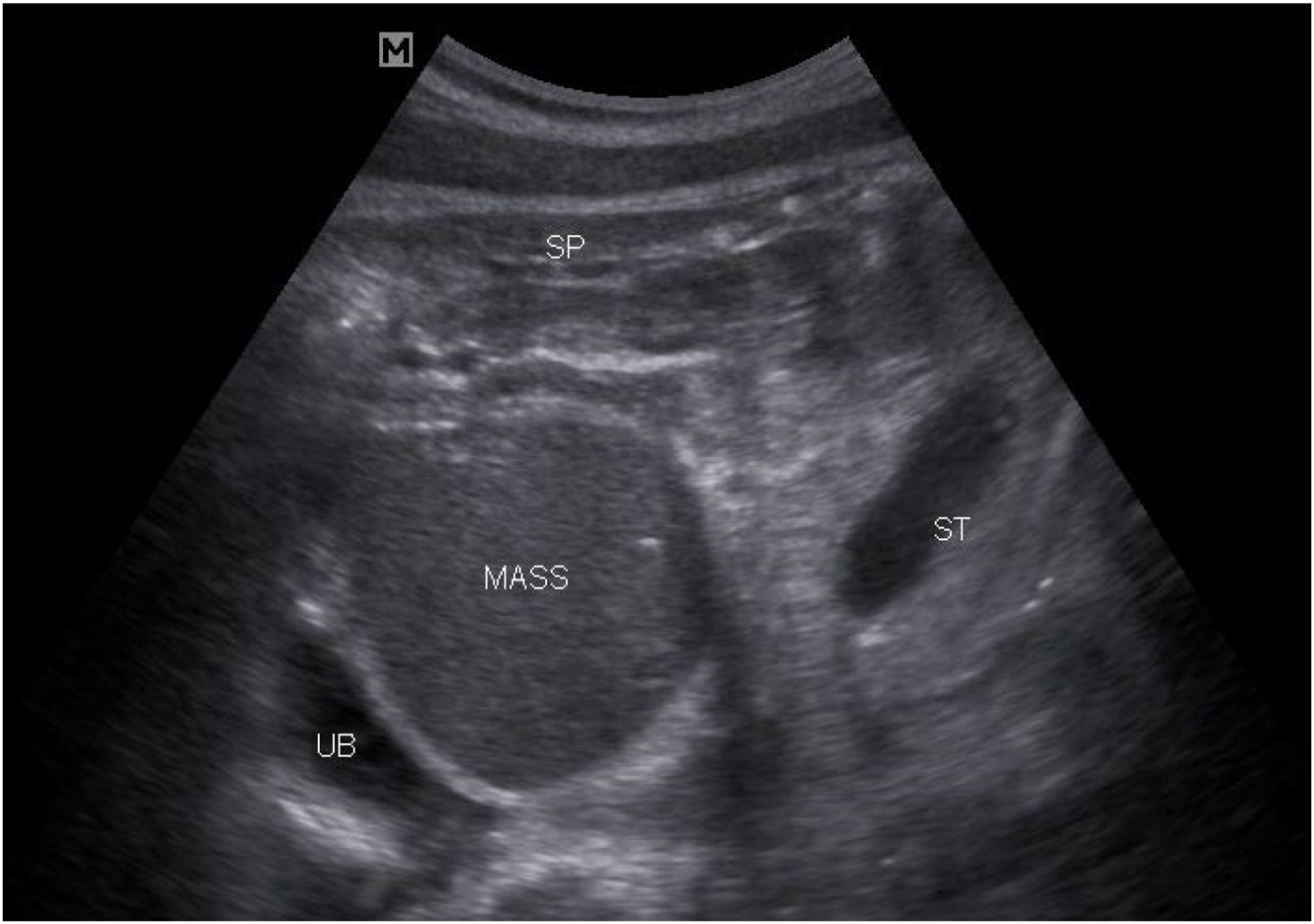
Antenatal ultrasound showing Huge retrovesical abdominopelvic cystic mass with internal echoes. UB, urinary bladder; ST, stomach; SP, spine.
